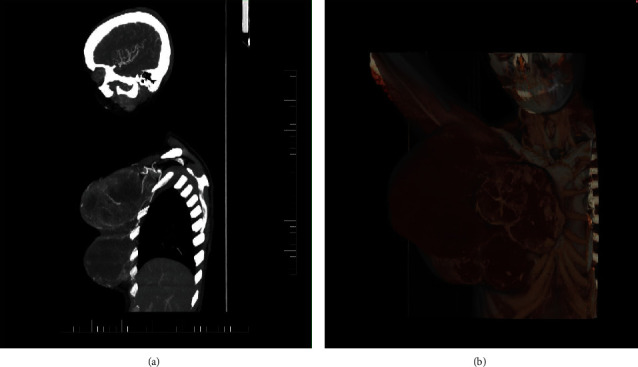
(a) Computer tomography (CT) arterial phase in the sagittal plane and (b) volume rendering technique with the fusion of venous phase. Partially seen magistral arteries and truncus costocervicalis feeding the tumor originating from the subclavian artery. The CT scan was performed during the last recurrence of the tumor.
